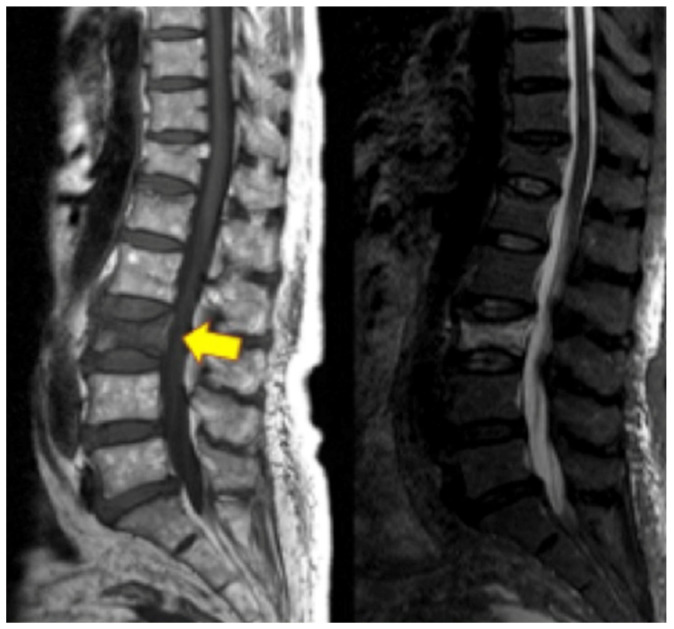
Lumbar spine MRI on the left with yellow arrowing pointing at the L3 vertebral compression fracture. On the right is an STIR image of the L3 vertebral compression fracture with hyperdense bone marrow changes representing acute vertebral compression fracture.
